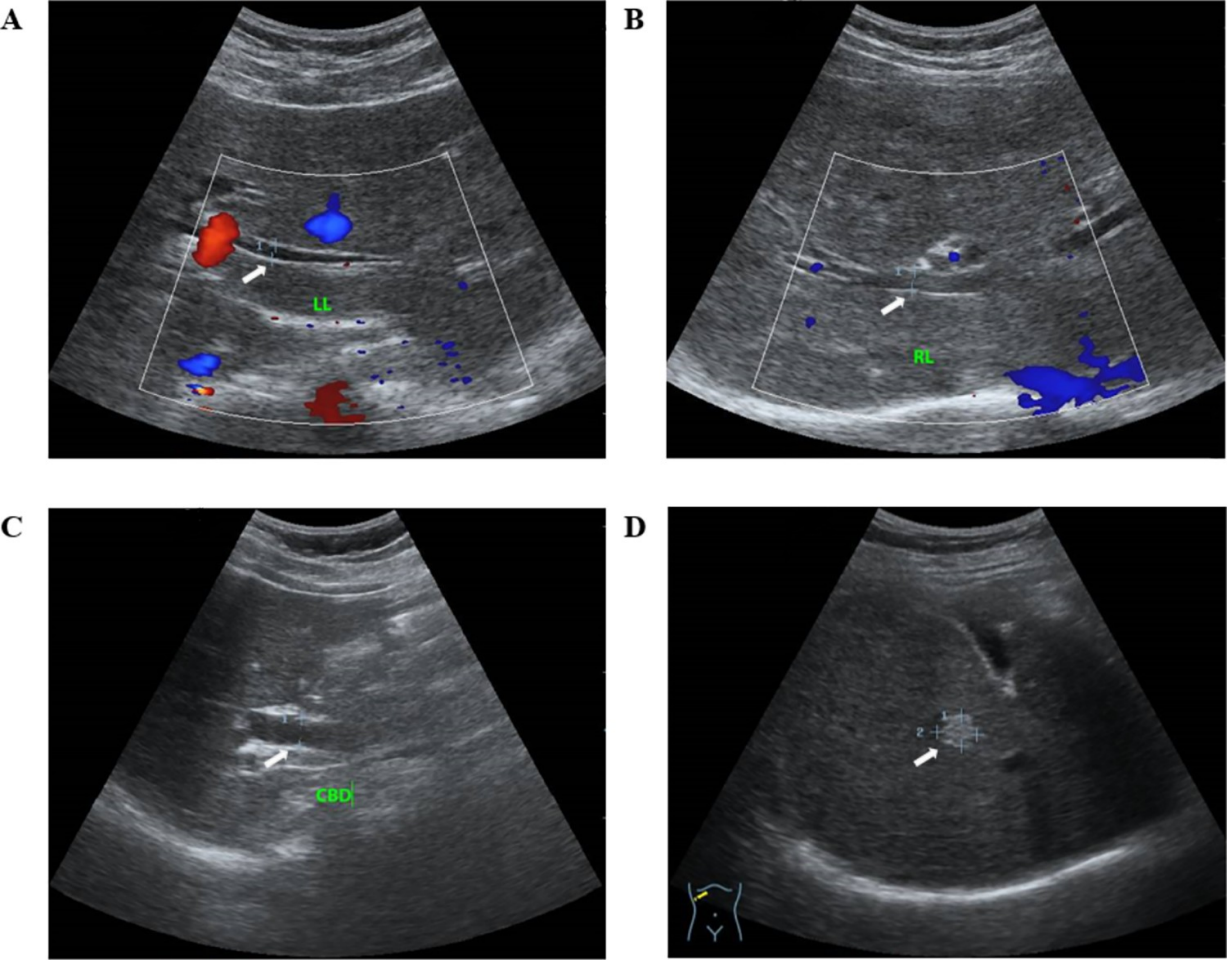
Ultrasound images for bile duct dilatation and liver masses. These ultrasound images, obtained from two different study participants (A-C from a 60-year-old male and D from a 63-year-old male), were classified into four categories for suspected CCA diagnosis: (A) left liver lobe (LL) dilatation (3.7 mm), (B) right liver lobe (RL) dilatation (4.8 mm), (C) common bile duct (CBD) dilatation (9.9 mm), and (D) a single hyperechoic liver mass with an ill-defined margin (9.3*11.9 mm). White arrows indicate areas of pathology, while red areas in A indicate blood flow toward the probe, and blue areas in A and B indicate blood flow away from the probe.
